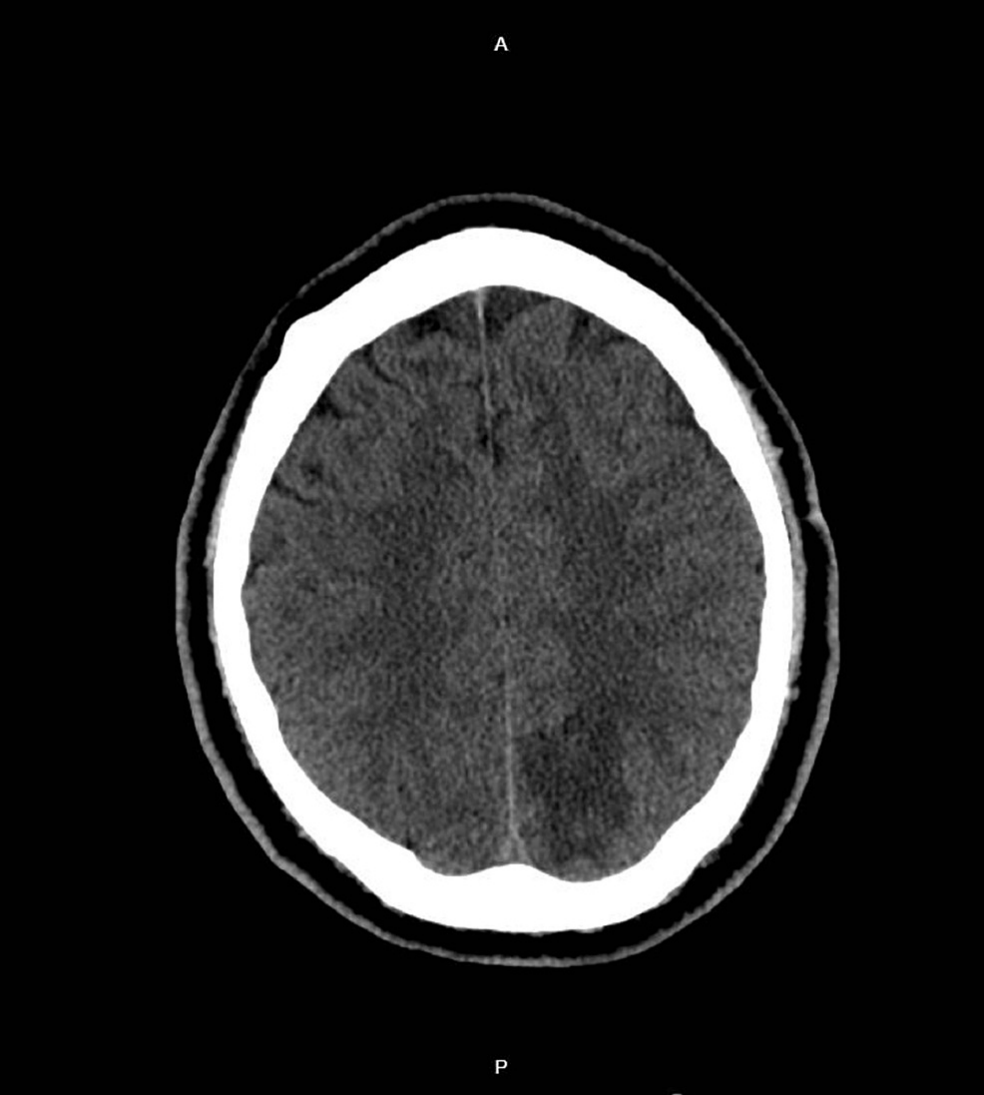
CT brain showing left occipito-parietal cortex hypodense area with loss of gray-white matter differentiation, suggestive of evolving stroke CT: Computerized tomography
